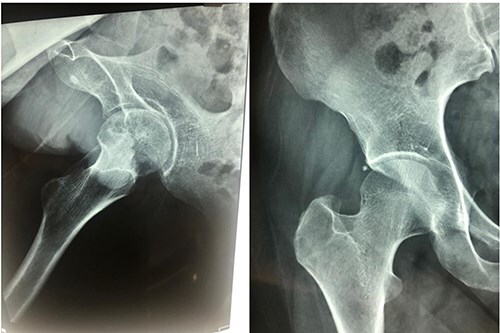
The preoperative radiographs showing moderate degree of osteonecrosis of femoral head.
